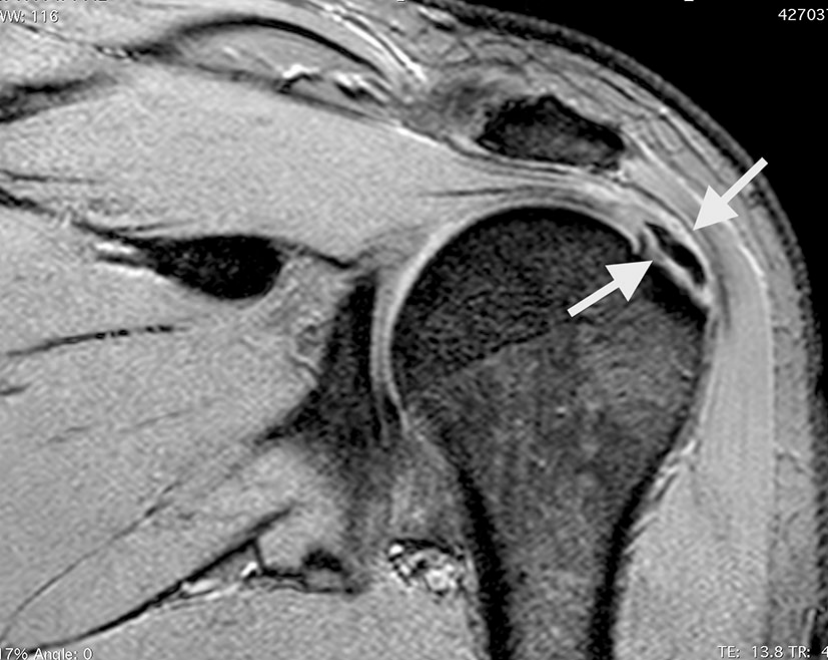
Coronal fatty suppressed MRI reveals a focus of chronic calcification with associated full-thickness supraspinatus tendon tear (*white arrows*)
